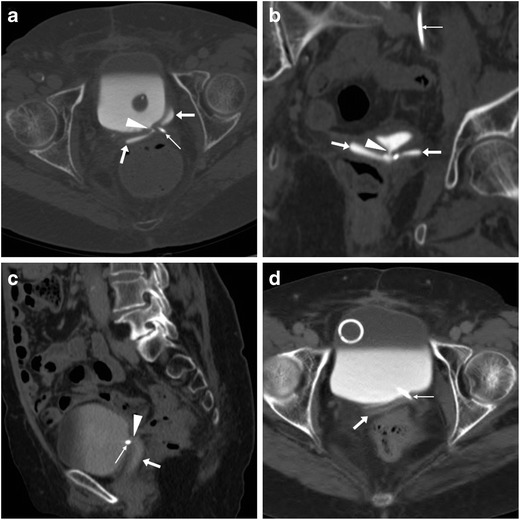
Vesico-vaginal fistula (VVF) developing after radical AH and radiotherapy for endometrial carcinoma in a 66-year-old female, manifesting with vaginal discharge without abnormal findings at gynaecological examination. Excretory-phase CT (A, B) showed a well- opacified urinary bladder with a Foley catheter and left-sided ureteral stent (thin arrow) in place, lateral retraction and opacification of the vagina (arrows) through a short communication (arrowheads) consistent with VVF. After vaginal electrocoagulation, long-term antibiotics and ureteral stenting, repeated CT urography 6 months later (C) showed persistent vaginal opacification (arrow) through the high-output supratrigonal VVF. A year later, CT follow-up (D) showed non-opacified vagina (arrow) indicating fistula closure (Partially reproduced with permission from ref. no. [62])
